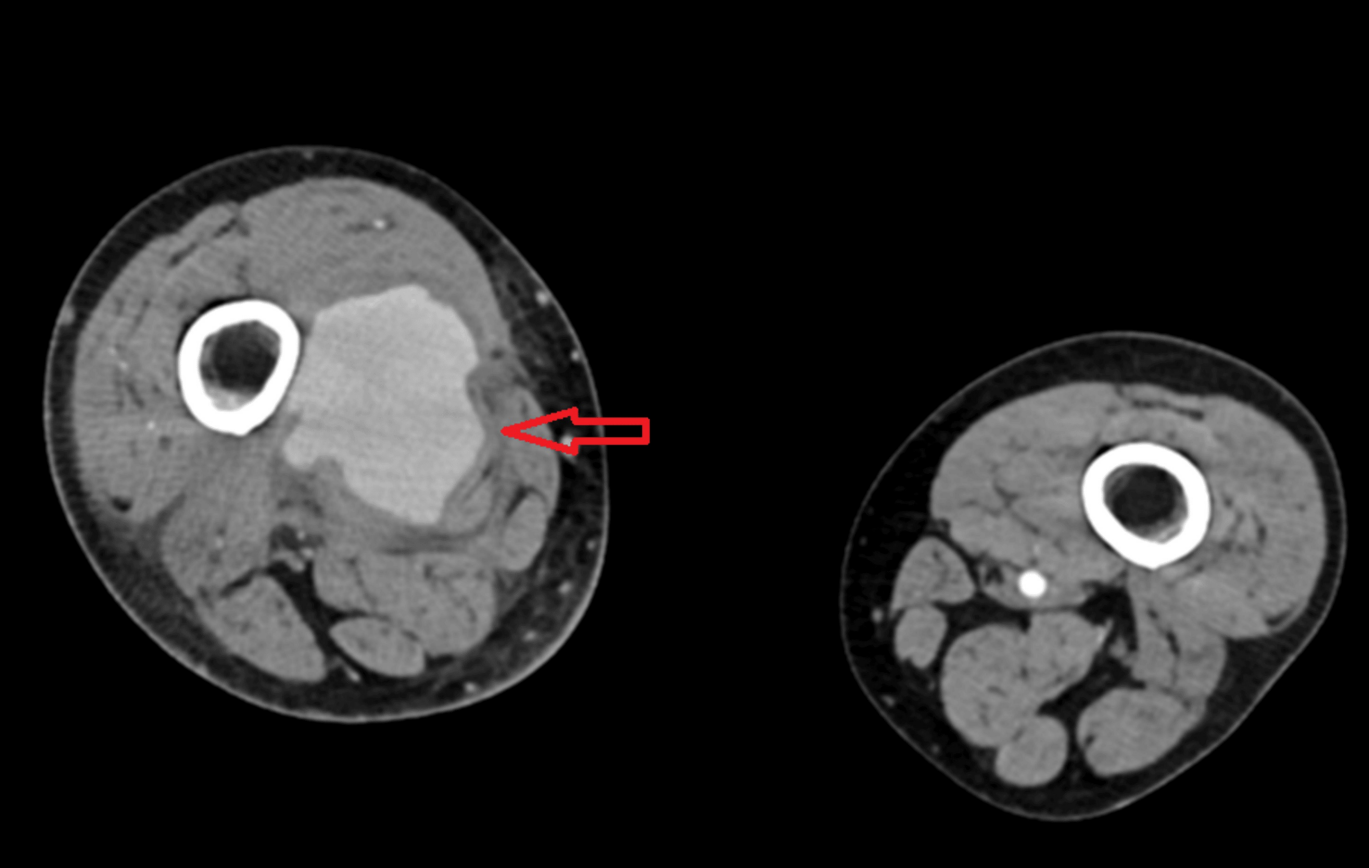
Axial CT angiogram of right superficial femoral artery aneurysm Contrast-enhanced axial CT angiography demonstrates a large, saccular aneurysm (red arrow) arising from the right superficial femoral artery, measuring approximately 6.4 × 6.3 × 7.3 cm in cross-section.
